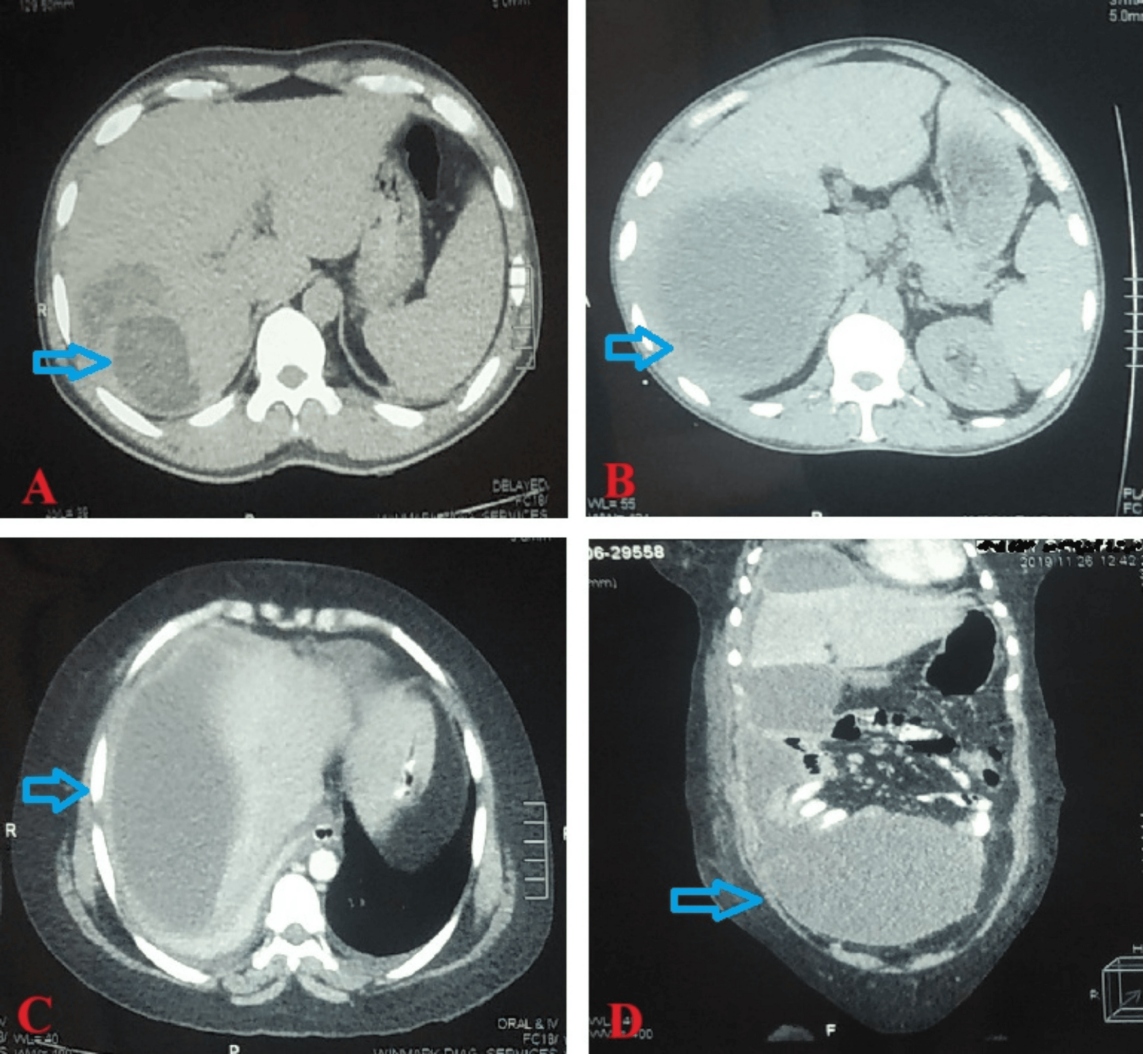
CT images of different liver abscess. (A) Small abscess (<5 cm) (axial section); (B) abscess between 5-10 cm (axial section); (C) large abscess (>10 cm) (axial section); (D) ruptured abscess with peritoneal dissemination (pointed arrow) (coronal section).
